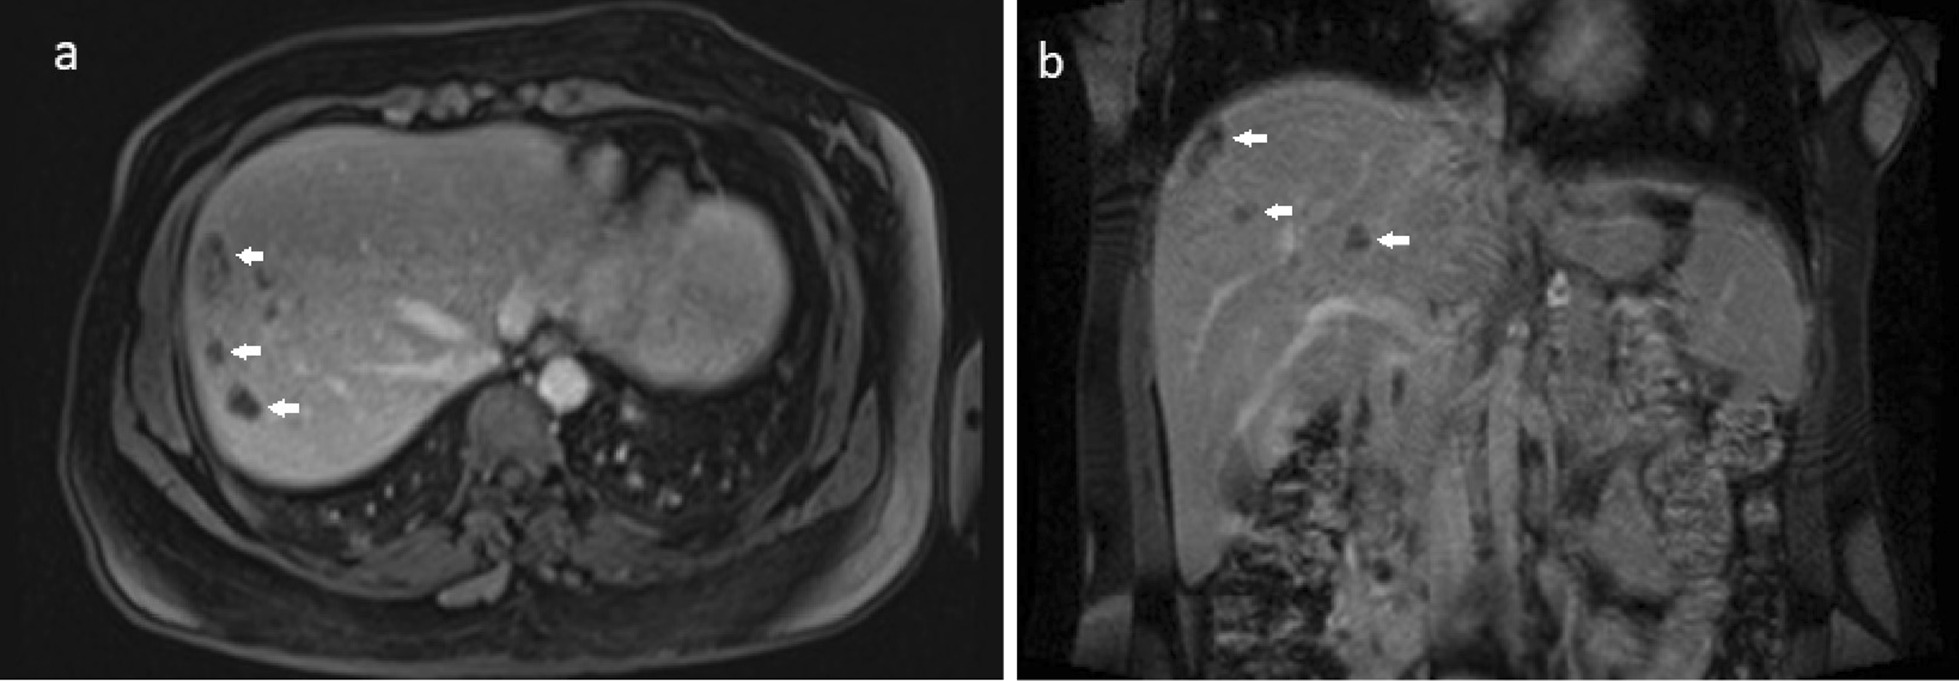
a A contrast-enhanced axial T1W MR image showing multiple, round, clustered hypointense subcapsular/parenchymal lesions with mild peripheral contrast enhancement in the liver (arrows). b A contrast-enhanced coronal T1W MR image showing multiple, round, clustered hypointense subcapsular/parenchymal lesions with mild peripheral contrast enhancement in the liver (arrows)
